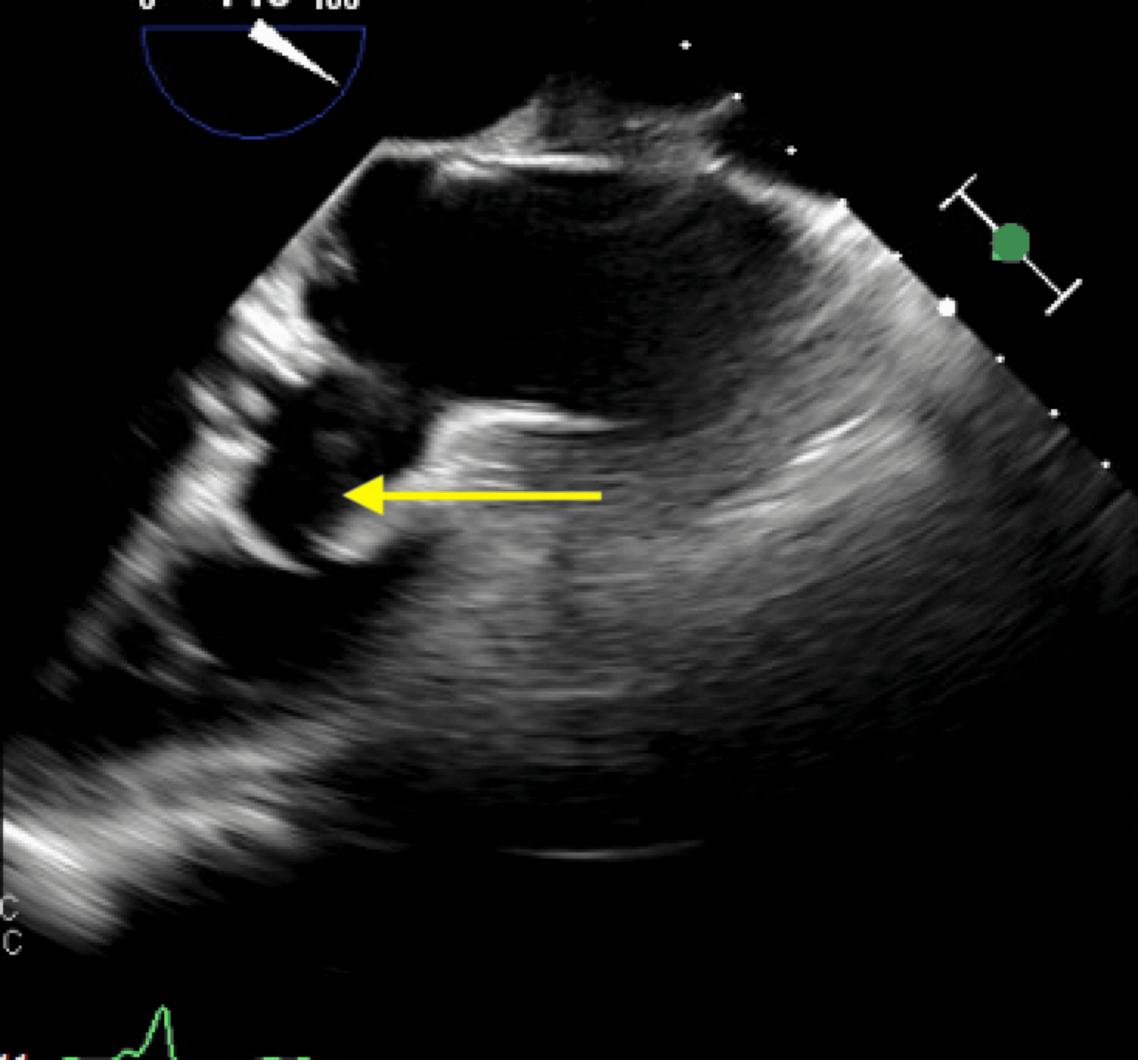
Transesophageal echocardiogram demonstrating a sinus of Valsalva aneurysm involving the right cusp (yellow arrow) (mid-esophageal aortic valve long-axis (ME AV LAX) view).
